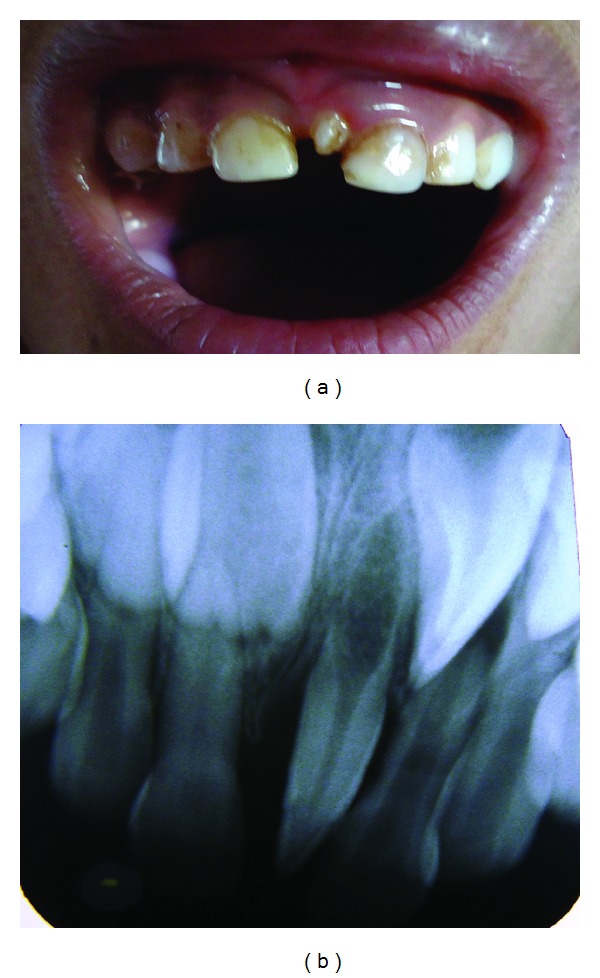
(a) Showing mesiodens in upper arch. (b) Radiograph showing rotated mesiodens and permanent incisor.
